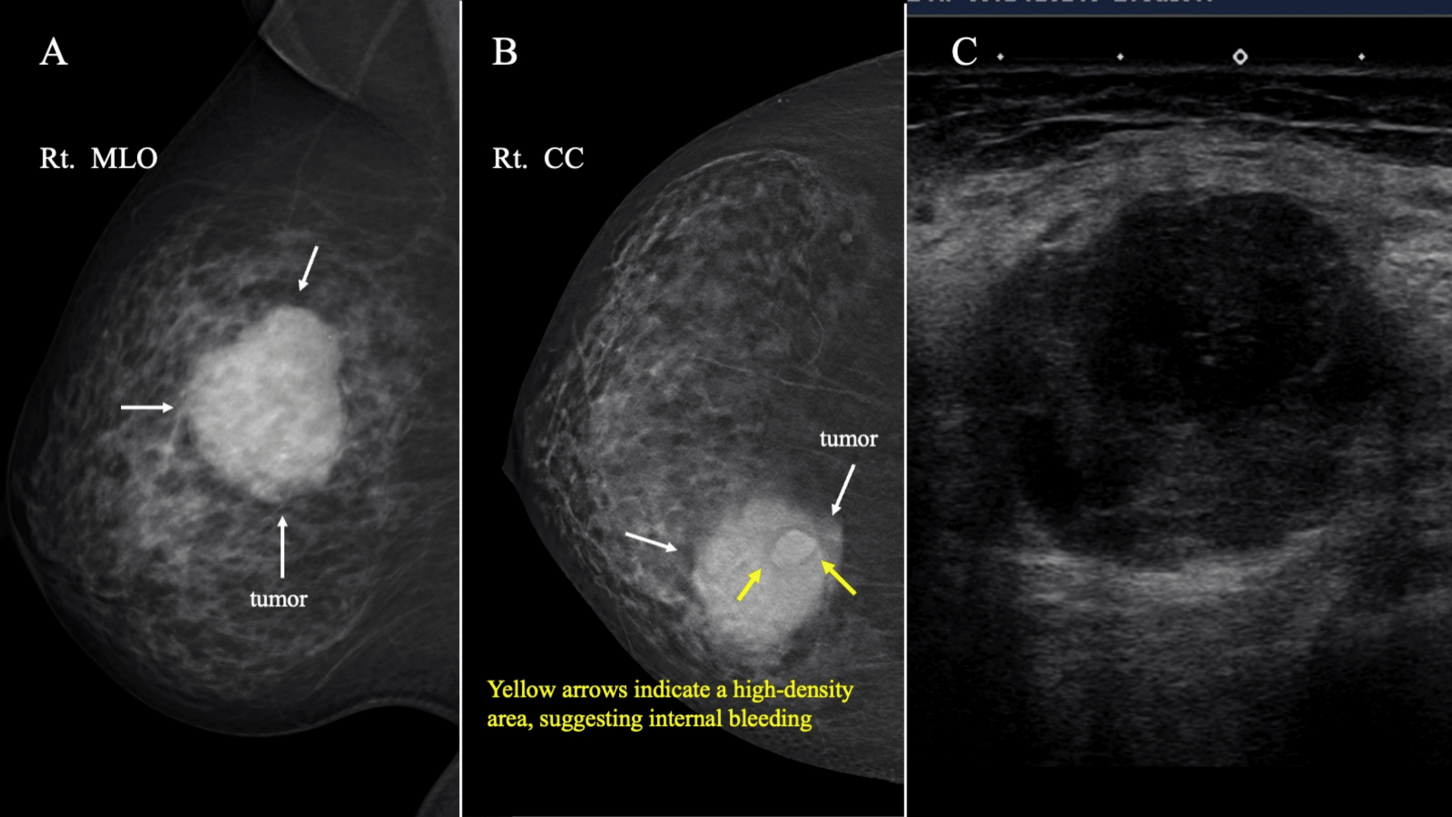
MG and US findings. (A) MG shows a lobulated, high-density mass (white arrow) on medio-lateral oblique view. (B) A craniocaudal view of 3D-MG showed a lobulated mass (white arrow) with relatively high-density area (yellow arrow) on the internal chest wall side of the tumor. The yellow arrows indicate a high-density area, suggesting internal bleeding. (C) US findings. US revealed a hypoechoic, heterogeneous tumor with an unclear border. MG: Mammography, US: Ultrasonography, 3D-MG: Tomosynthesis.
